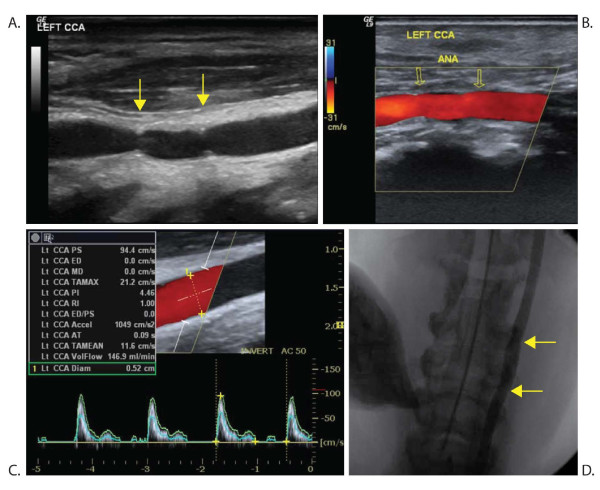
Ultrasound and angiography of a common carotid artery with vein graft. Gray-scale ultrasound (A) and angiography (D) showing the anatomy. Color Doppler (B) and spectral Doppler (C) investigation showing normal flow. Yellow arrows point at the anastomoses (ANA). CCA = common carotid artery. PI = pulsatility index. RI = resistive index.
